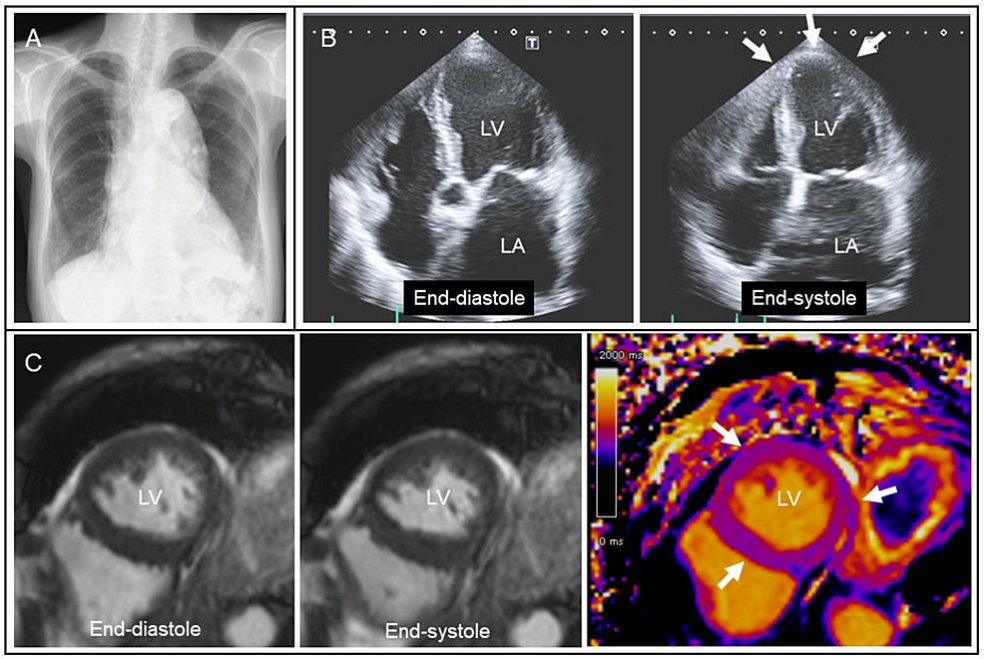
Cardiac examinations during the first hospitalization Chest radiography on the first admission showed enlarged aortic and cardiac silhouettes (A). Transthoracic echocardiography revealed diffuse LV hypokinesia including apical area with an ejection fraction of 30% (B, arrows). Cine cardiac magnetic resonance also showed diffuse LV hypokinesia with normal T1 mapping measurements (C, arrows).
